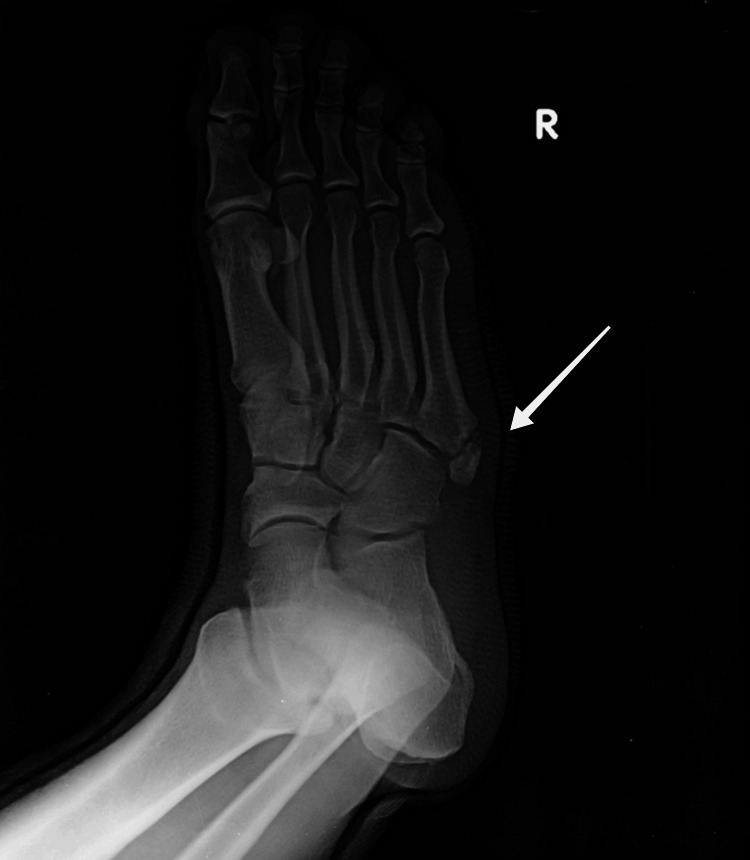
Right foot oblique radiograph. The white arrow points at OVP proximal to the fifth metatarsal base. The differential for a bony opacity in this region is an unfused apophysis orientated parallel to the cortex of the metatarsal base OVP: os vesalianum pedis
